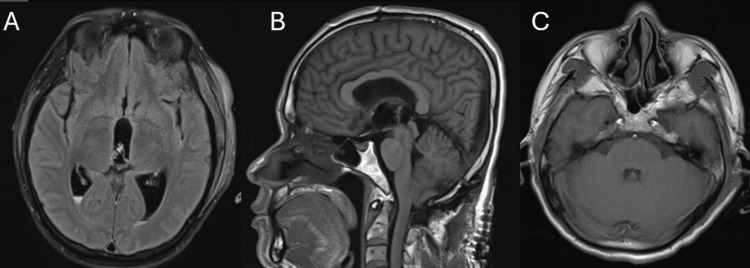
MRI of the brain on hospital day 12 following the removal of a 1.5 cm cyst from the fourth ventricle. A: Axial T2 fluid-attenuated inversion recovery imaging showing small-volume postoperative intraventricular hemorrhage without evidence of residual cysts. B: Sagittal T1-weighted image showing no evidence of residual cysts. C: Axial T1 image showing no evidence of residual intraventricular cysts (Figure 2).
